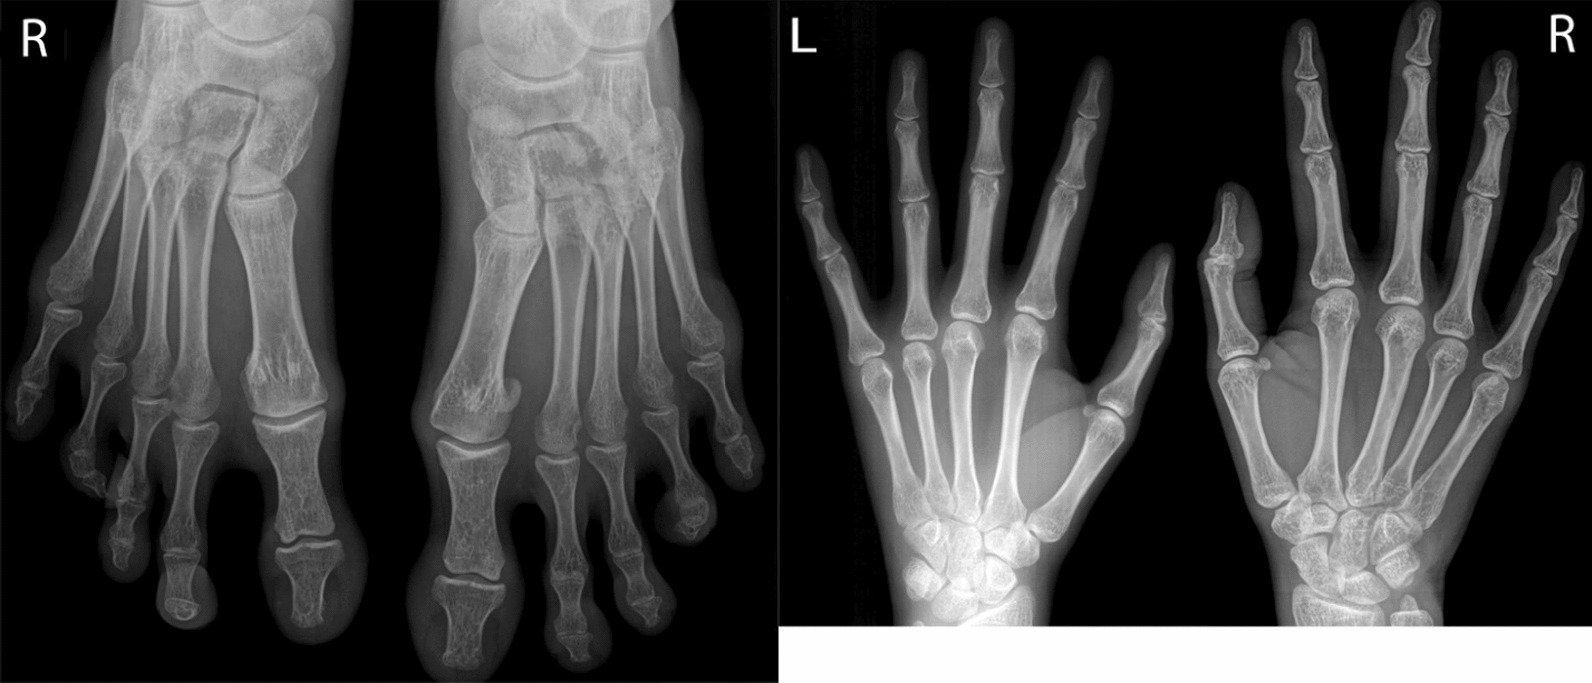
Foot X-ray and hand X-ray
